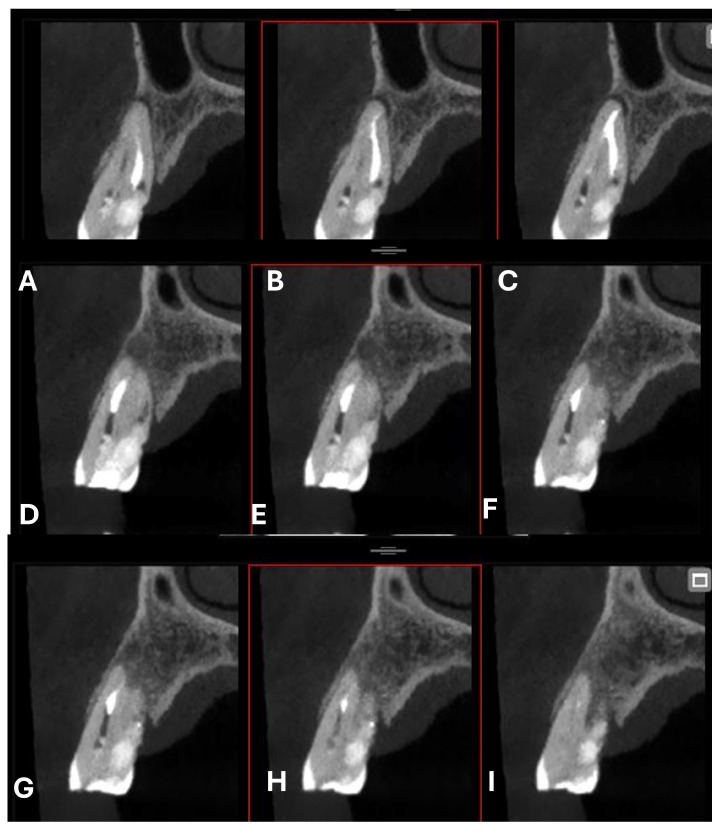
Cross-section CBCT images of tooth 1.4 after six months of healing. (A–I) Sequential scrolling of EPL healing.
